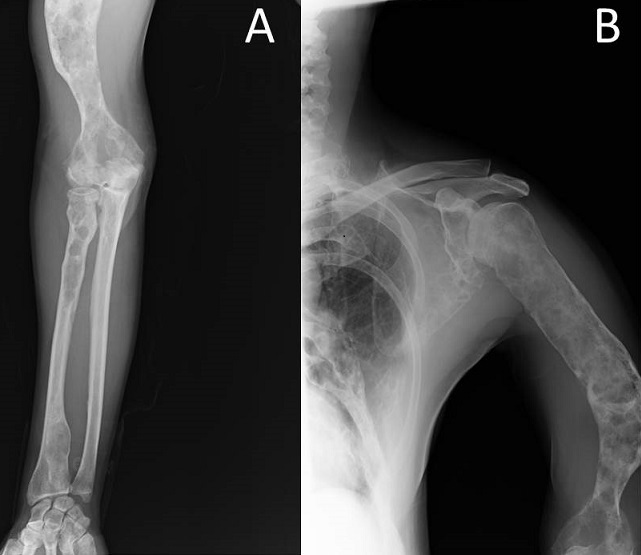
A) Radiographie de face montrant une dysplasie fibreuse du tiers proximal et distal du radius; B) Radiographie de face objectivant des lésions de dysplasie fibreuse intéressant le col de l'omoplate et la totalité de l'humérus
